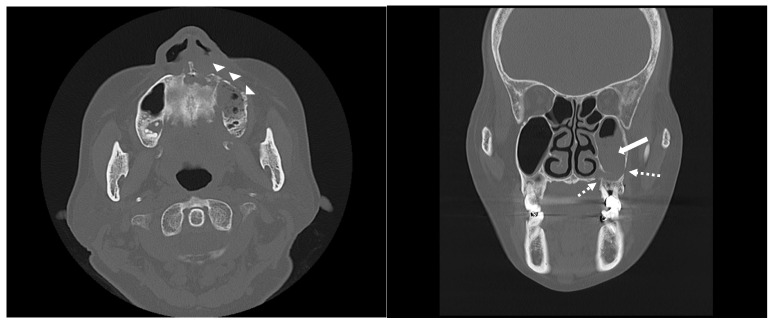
Axial (left) and coronal (right) CT images reveal pathological fracture (dotted arrows) and ill-defined osteolytic lesion (arrow heads) with maxillary sinusitis (arrow) limited to the left side of maxilla.
